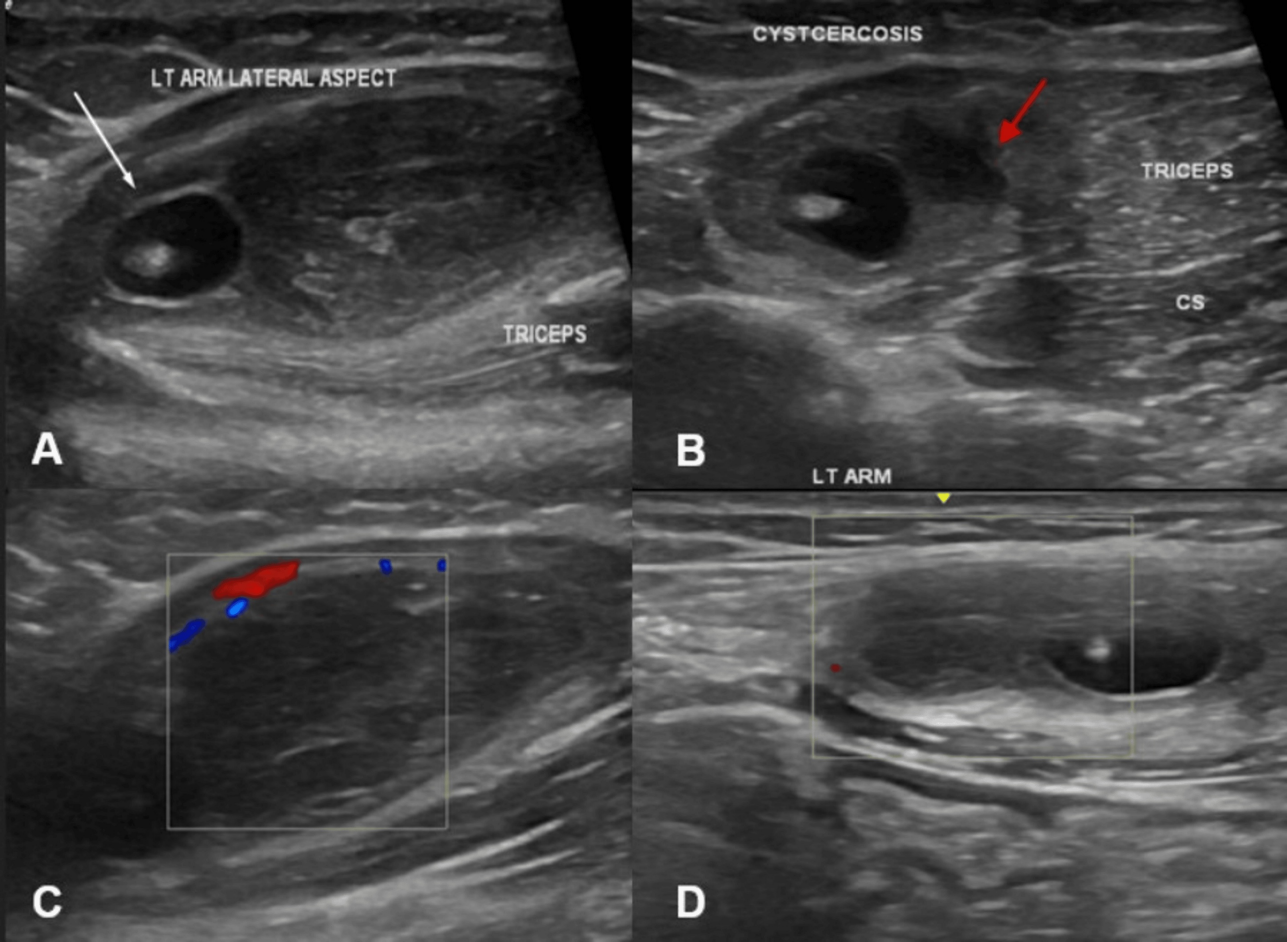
Ultrasound of left arm showing intramuscular abscess in triceps muscle, along the direction of longitudinal fibers of the muscle Ultrasound images of the left arm in longitudinal section (A) and cross-section (B) showing a well-defined cystic lesion with an eccentrically placed echogenic nodule (indicated by white arrow) and surrounding intramuscular abscess (indicated by red arrow) seen in the triceps muscles. Color Doppler images in longitudinal section (C) and cross-section (D) showing mildly increased peripheral vascularity around the hypoechoic area - likely surrounding inflammatory changes.
